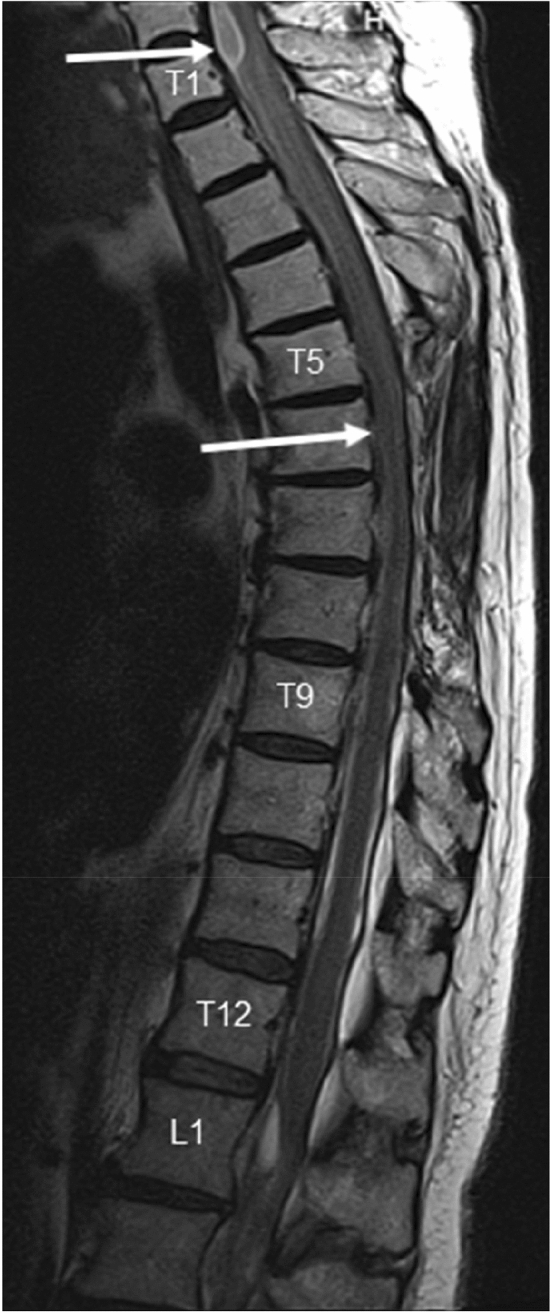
MRI T2-weighted STIR-sagittal view of spine board strict adhesion of the spinal cord to the dura mater in the thoracic spine with complete reduction of front fluid collection of the thecal sac. Numerous adhesions of pia mater with cystic lesions in the front part of the spinal canal at the cervicothoracic and thoracolumbar junction
